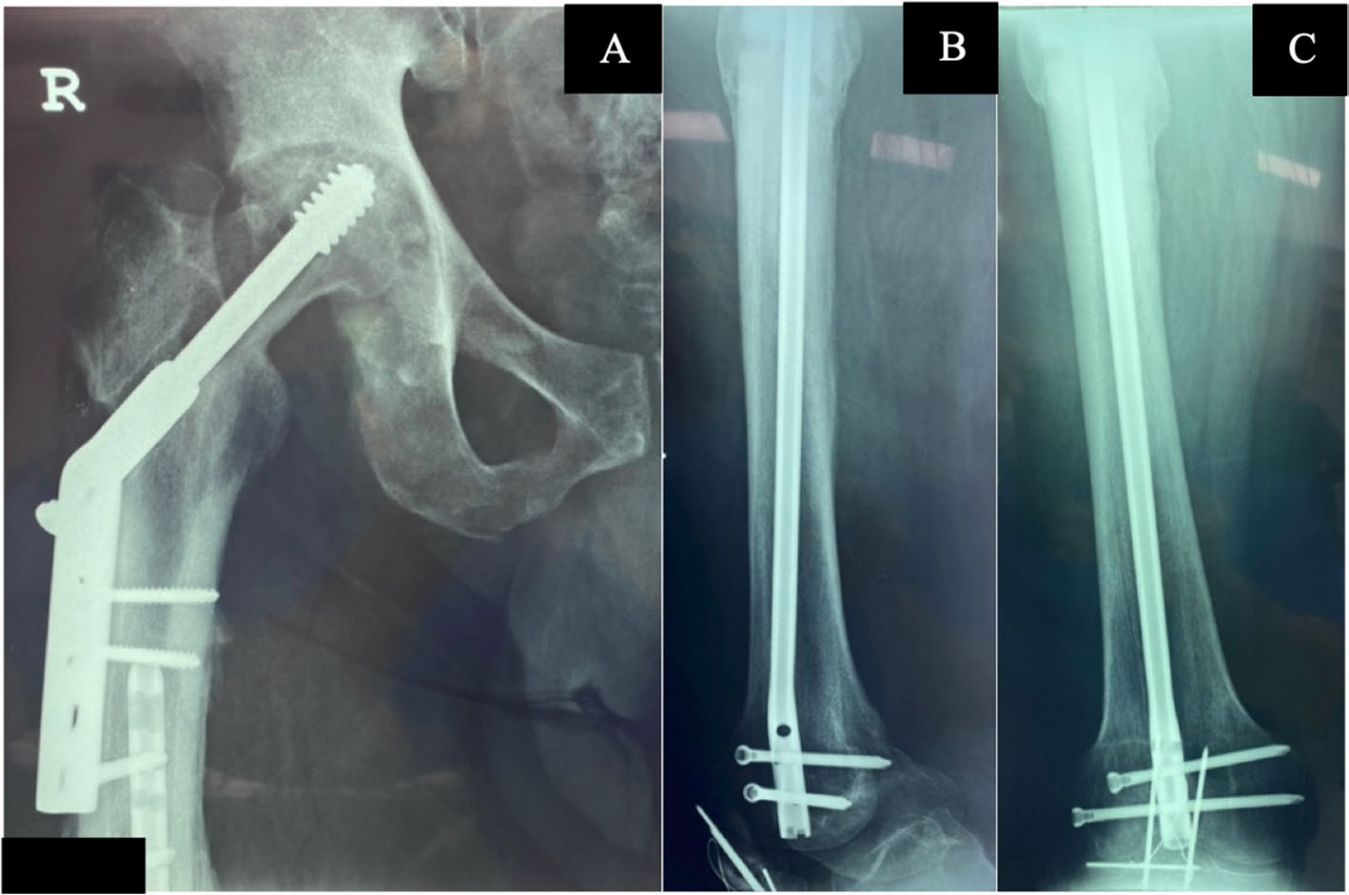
Anteroposterior radiograph of the hip (A) shows cut out of the lag screw in the DHS. Complete union of the associated femoral shaft fracture in both the lateral (B) and anteroposterior (C) radiographs with the retrograde femoral nail in-situ
